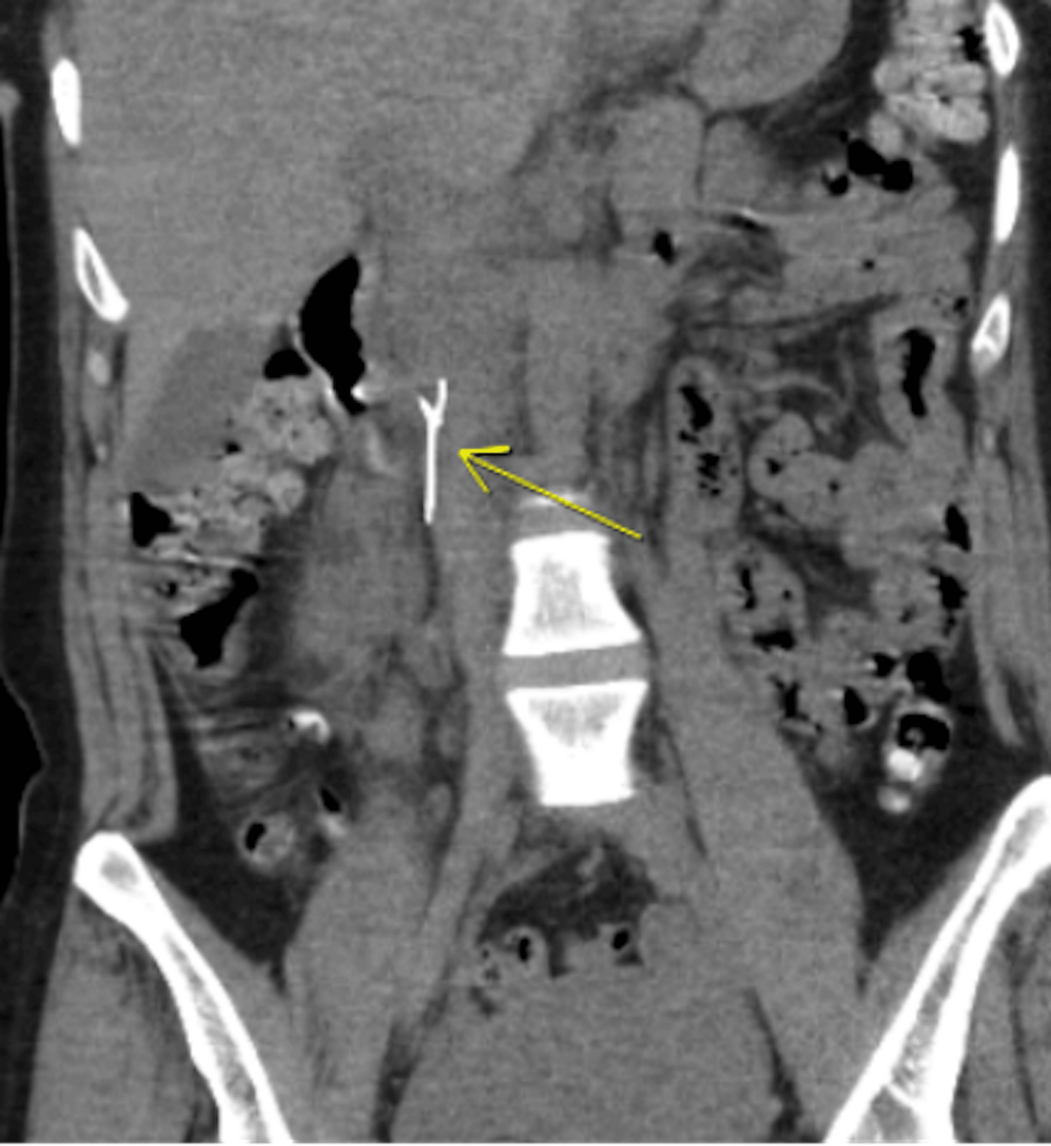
CT abdomen displaying linear metallic densities, consistent with retained IVC filter fragment.
